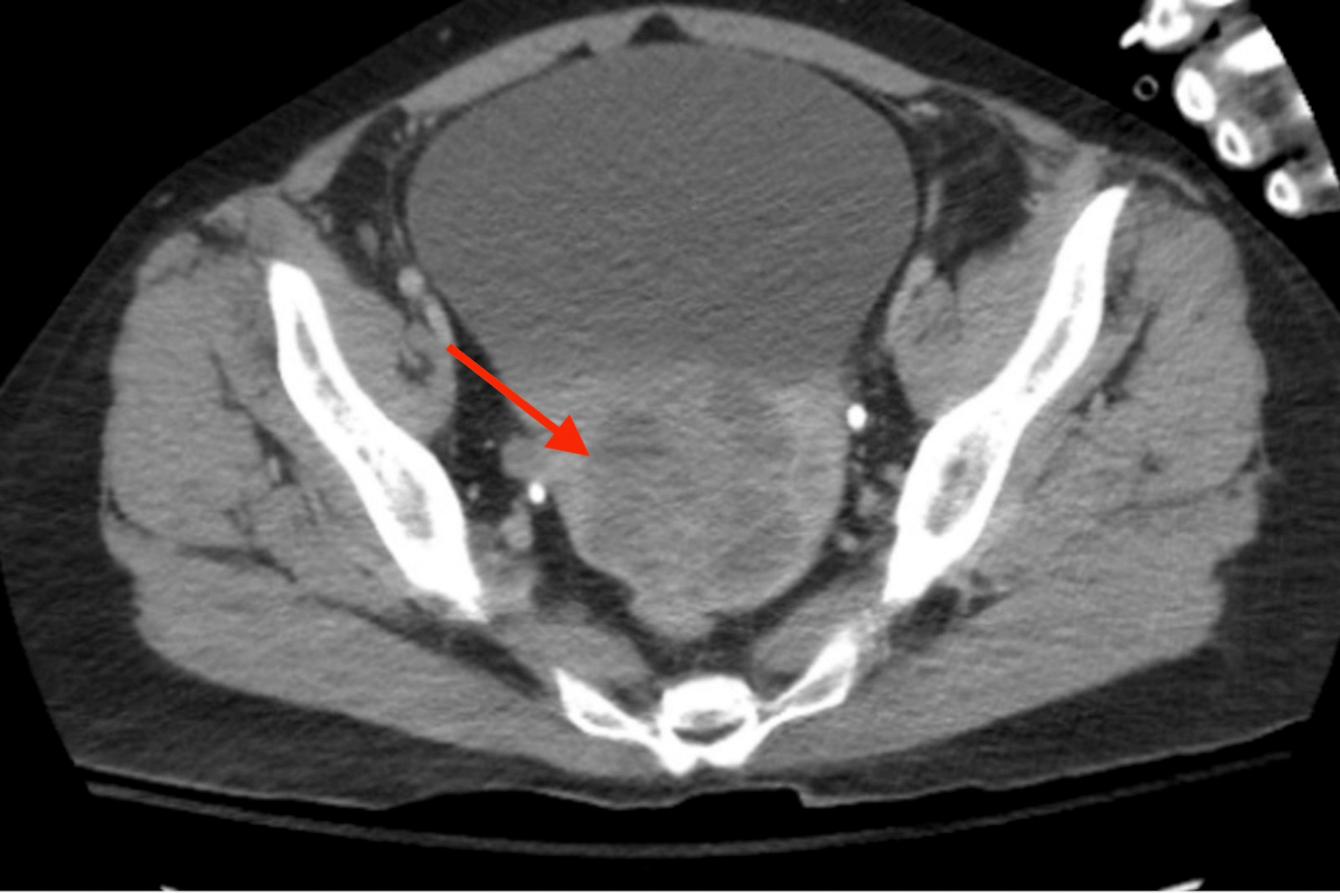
Axial CT abdomen and pelvis Heterogeneous irregular mass present within the uterus.
